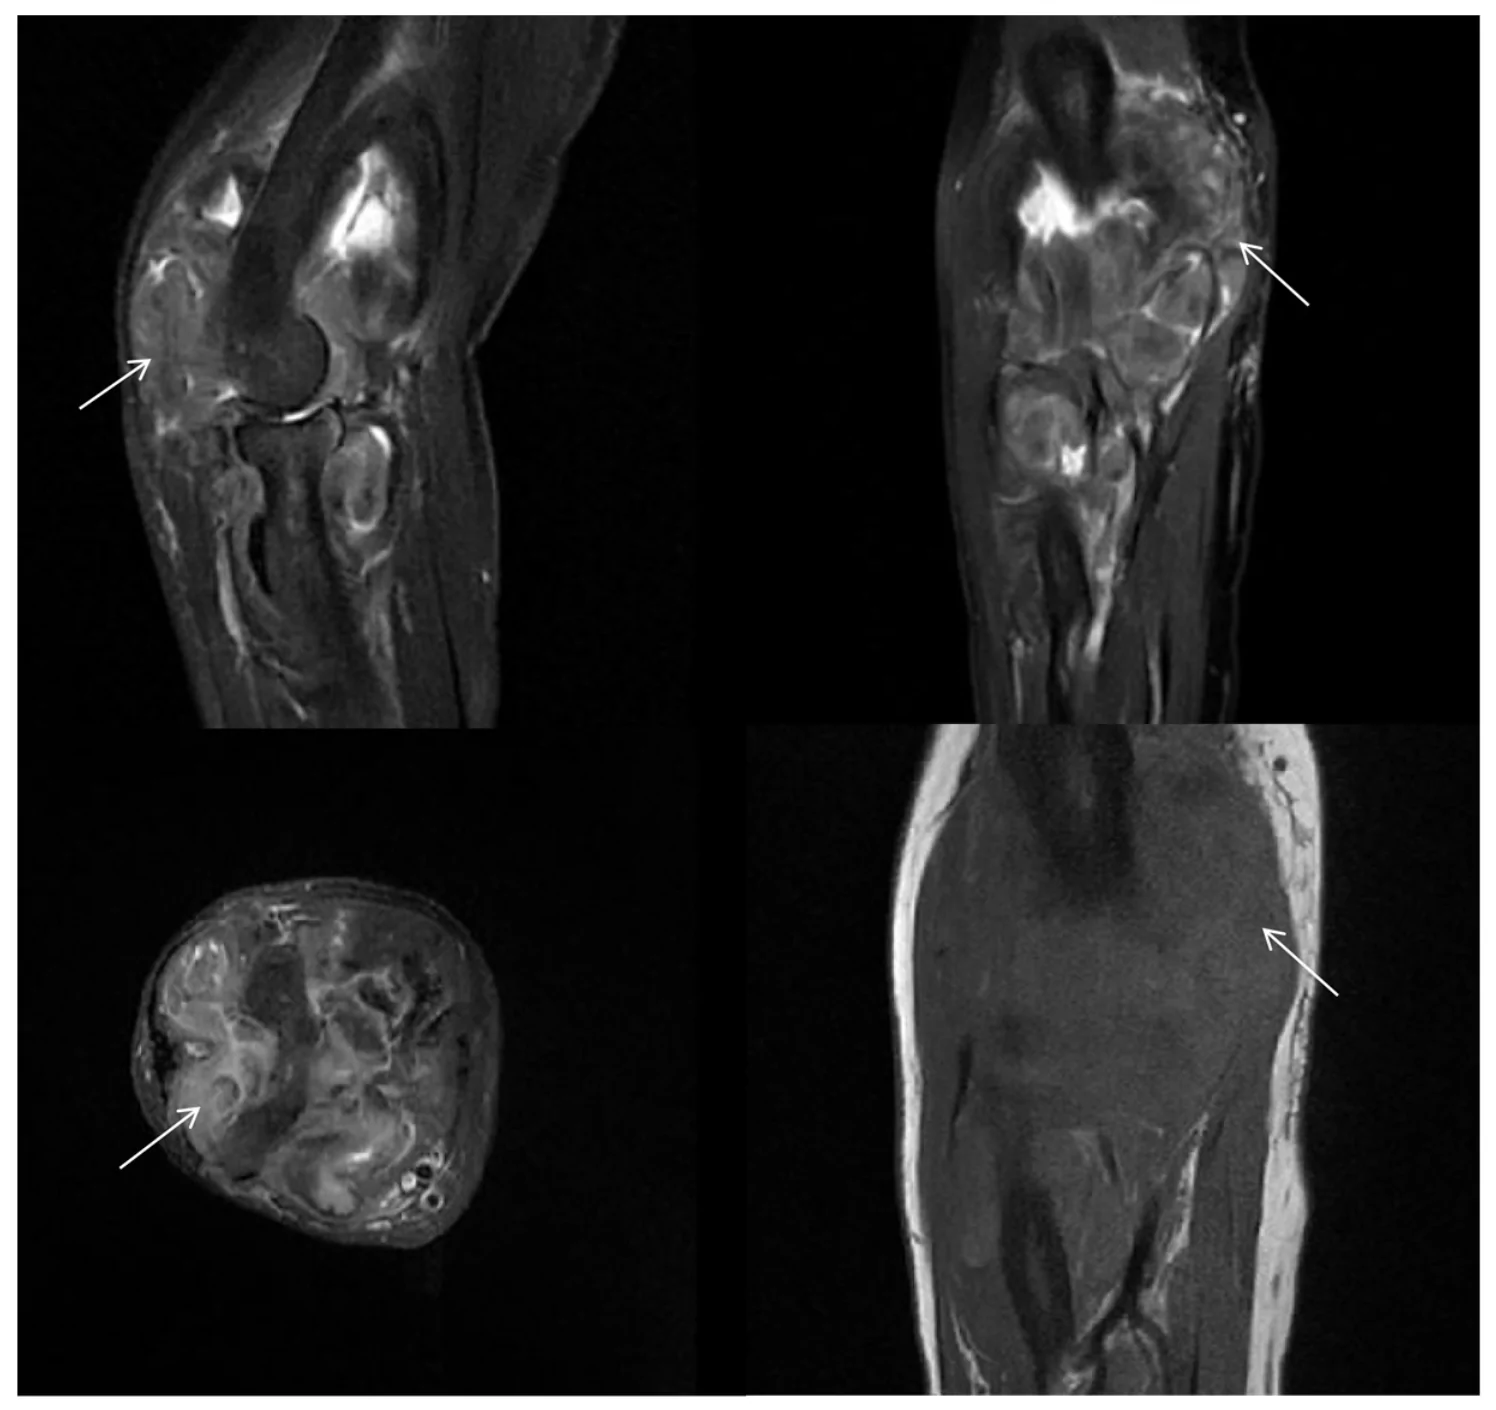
MRI findings of the right elbow lesion. Multiplanar MRI demonstrates an ill-defined infiltrative periarticular soft-tissue lesion around the right elbow, with heterogeneous signal intensity and adjacent osseous erosion. The arrows indicate the location of the lesion.
